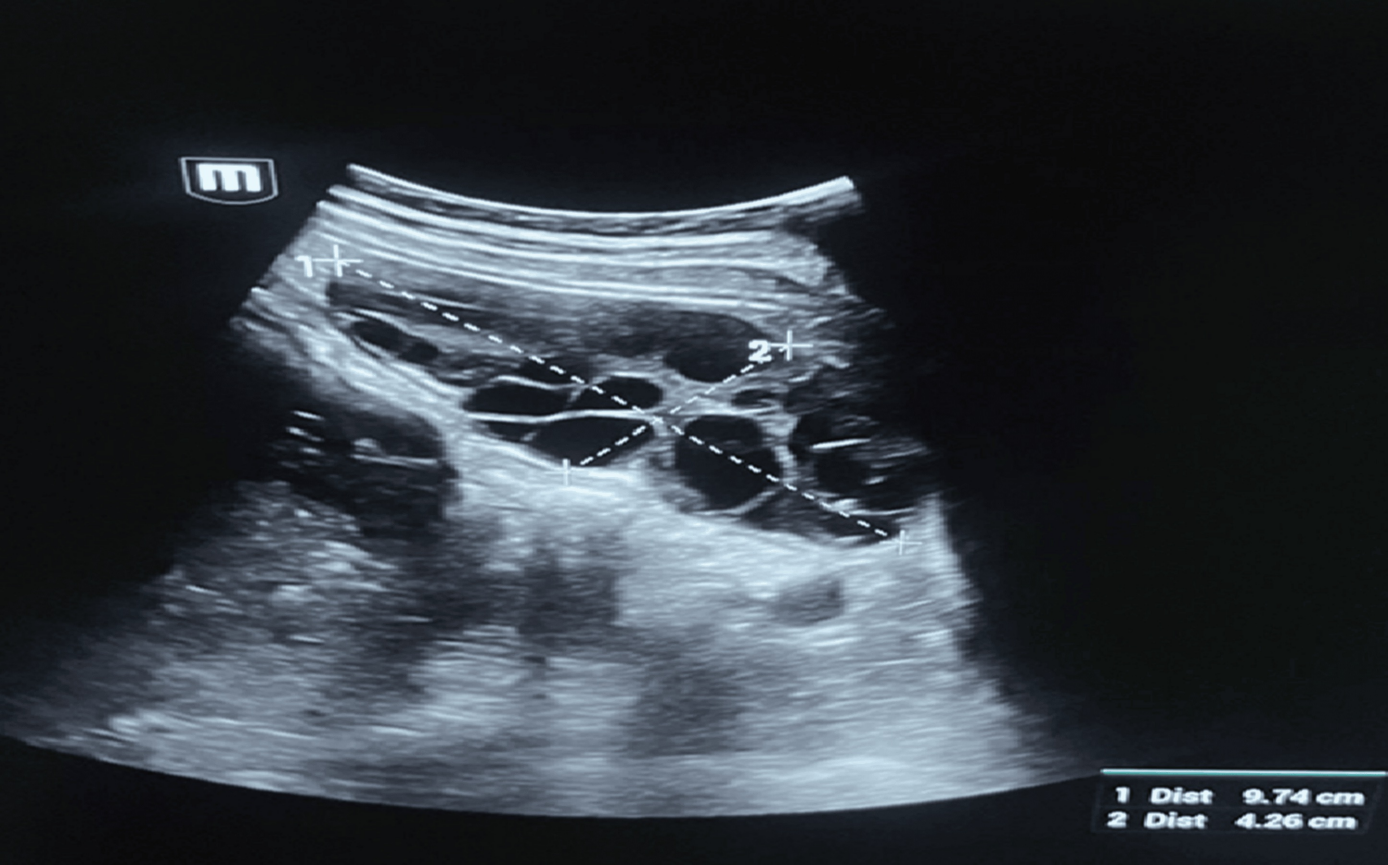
Ultrasound image of the enlarged ovary
